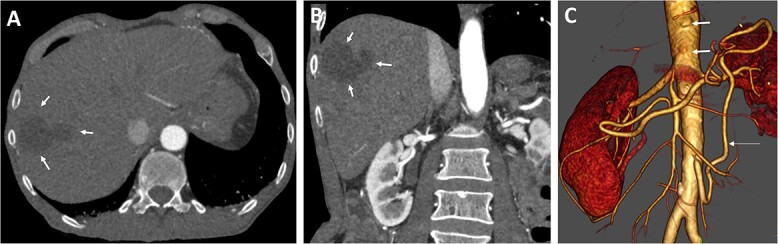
Preoperative axial (A) and coronal (B) CT angiographic images demonstrate hypoenhancement of all of the hepatic parenchyma and hypodense, hepatic lesion measuring 48 × 47 × 34 mm in the segment V and VI (arrows). (C) 3D reconstructed CT angiography shows occlusion of the celiac and superior mesenteric arteries (thick arrows) and hypertrophy of the inferior mesenteric artery and artery of Drummond (thin arrow).
